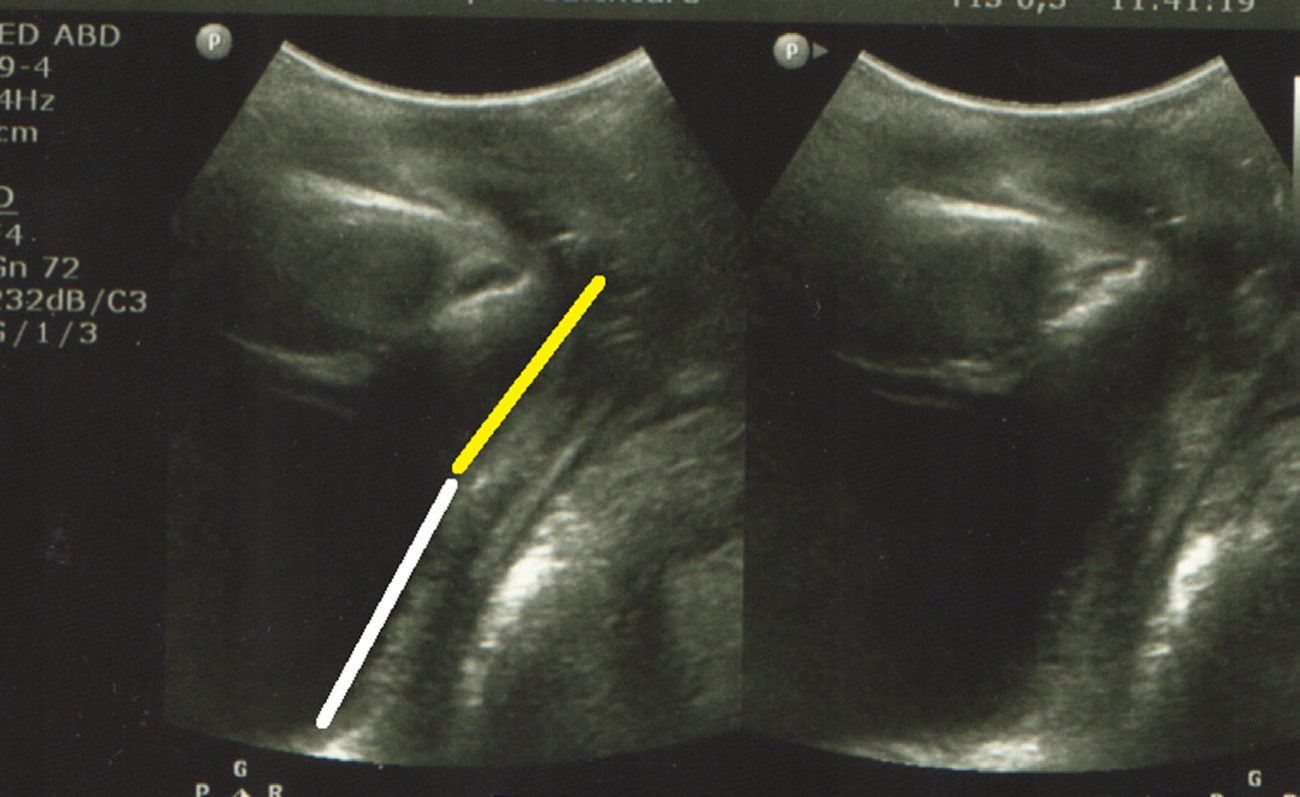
Perineal ultrasound: flat vesical urethral angle (parallel lines), Yellow line—posterior wall of the urethra and white line–posterior wall of the bladder.
